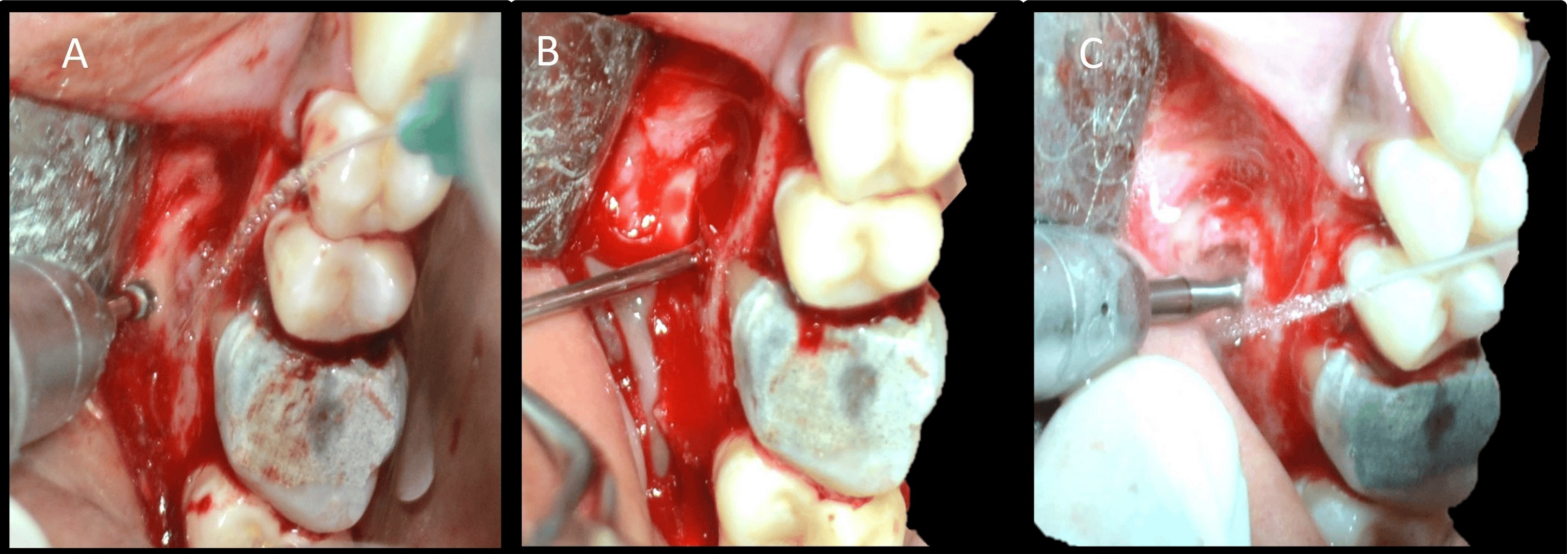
Osteotomy and root end resection A: Osteotomy, B: Periapical curettage, C: Root end resection of the mesiobuccal root
